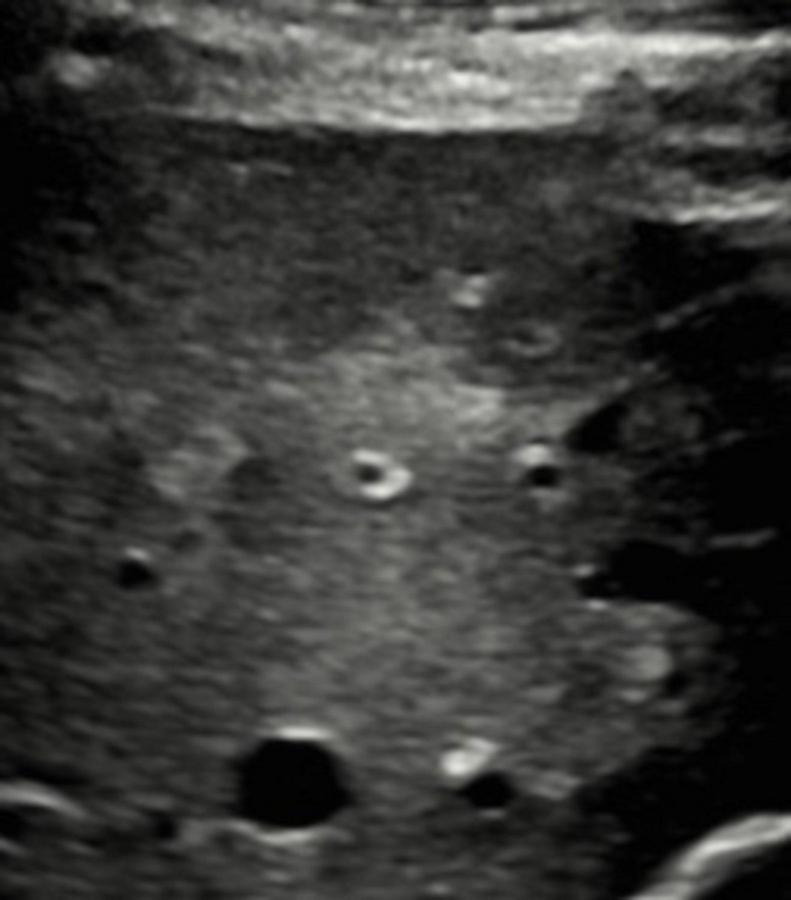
Follow up ultrasound of the liver no longer showing abscess. Four months follow up right upper quadrant ultrasound demonstrates interval resolution of the central hepatic lesion.
